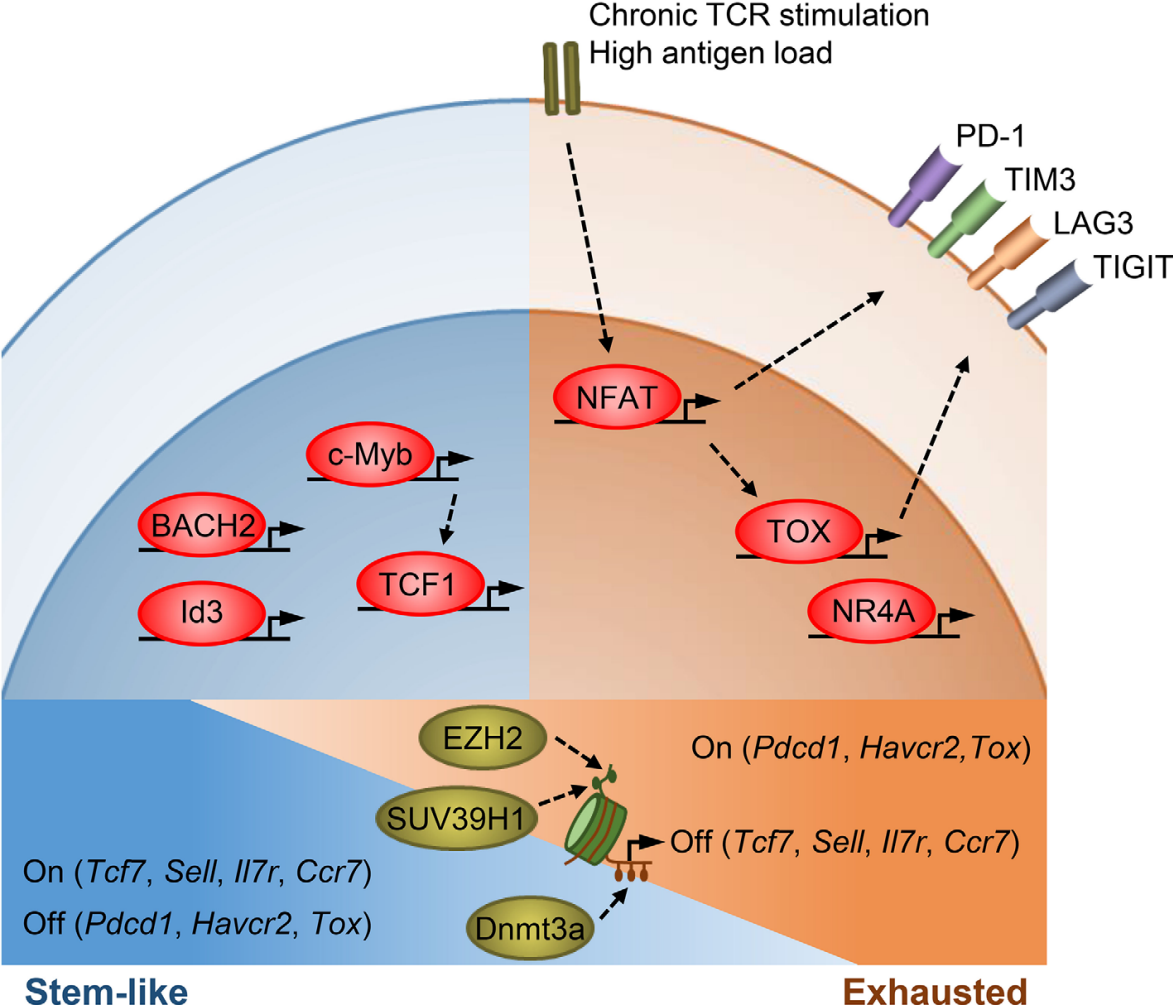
Transcriptional and epigenetic regulation of T cell stemness and exhaustion. Transcription factors TCF1, BACH2, Id3, and c-Myb promote memory T cell formation as well as stemness in T_PEX_ cells. TCF1, BACH2, and c-Myb also restrain effector T cell differentiation. c-Myb is a transcriptional activator of *Tcf7*. Under chronic antigen exposure or high antigen load, the TCR-NFAT-TOX/NR4A axis drives the exhaustion program of T cells and promotes the expression of multiple inhibitory receptors. TOX/TOX2 and NR4As are secondary transcription factors induced by initiating NFAT. Epigenetic mechanisms, such as histone modifications and DNA methylation, act in concert with transcription factors to regulate T cell states. SUV39H1 and EZH2 silence stem/memory genes during effector T cell differentiation by methylating H3K9 and H3K27, respectively. Dnmt3a mediates *de novo* DNA methylation to inhibit the expression of stem/memory genes during effector differentiation. T_EX_ cells have an epigenetic profile distinct from that of T_EFF_ and memory T cells, though how epigenetic enzymes regulate the expression of exhaustion genes remains unclear.
